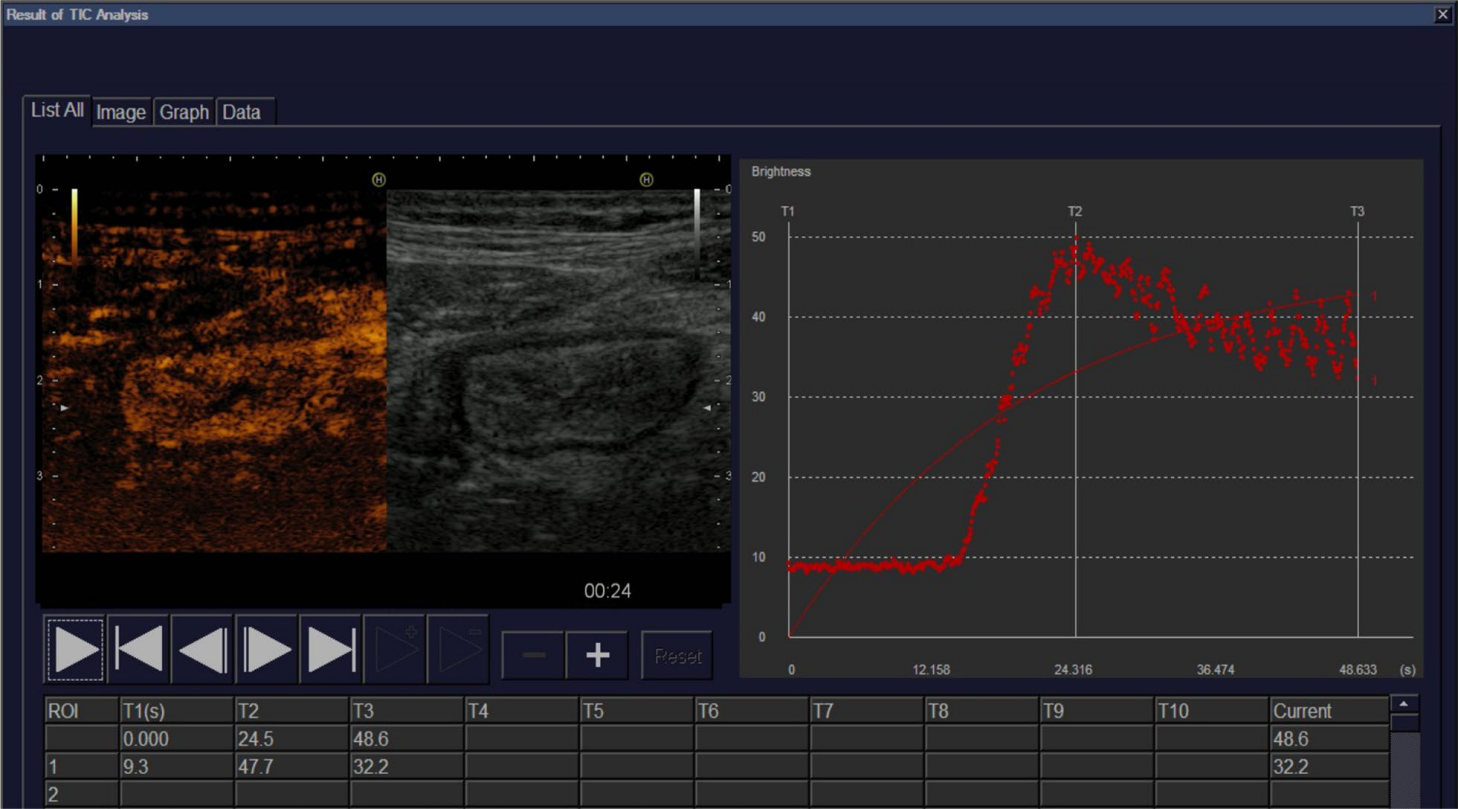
Contrast-enhanced ultrasound and peak intensity curve examination
